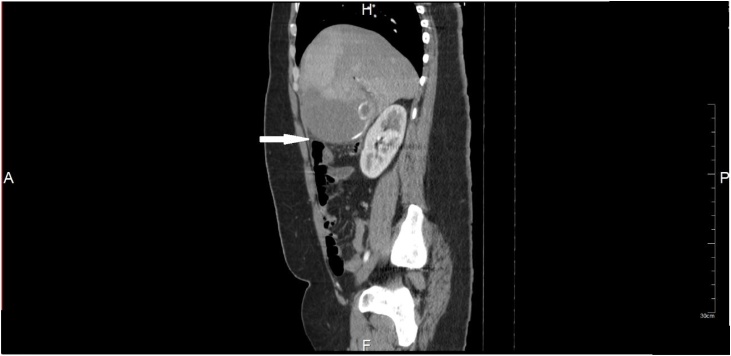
Sagittal view of CT scan.
